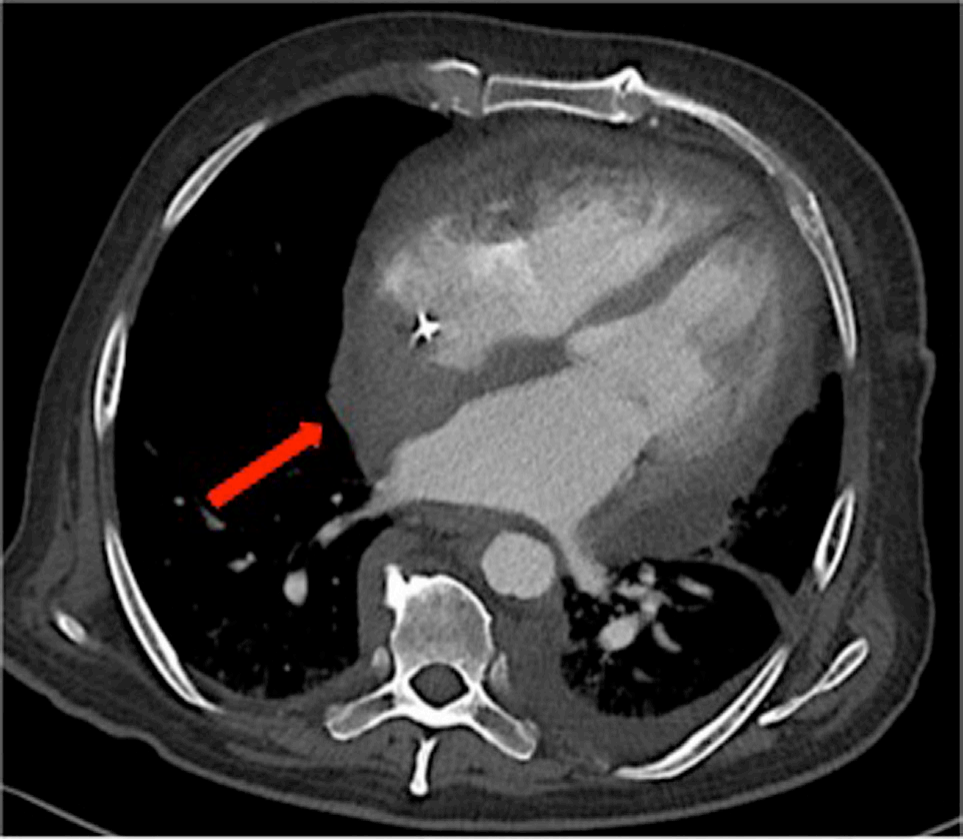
A contrast-enhanced computed tomography showing thickening of the pericardial sheets, pericardial effusion and a pseudo-tumoral infiltration of the right atrium [*red arrow*].
